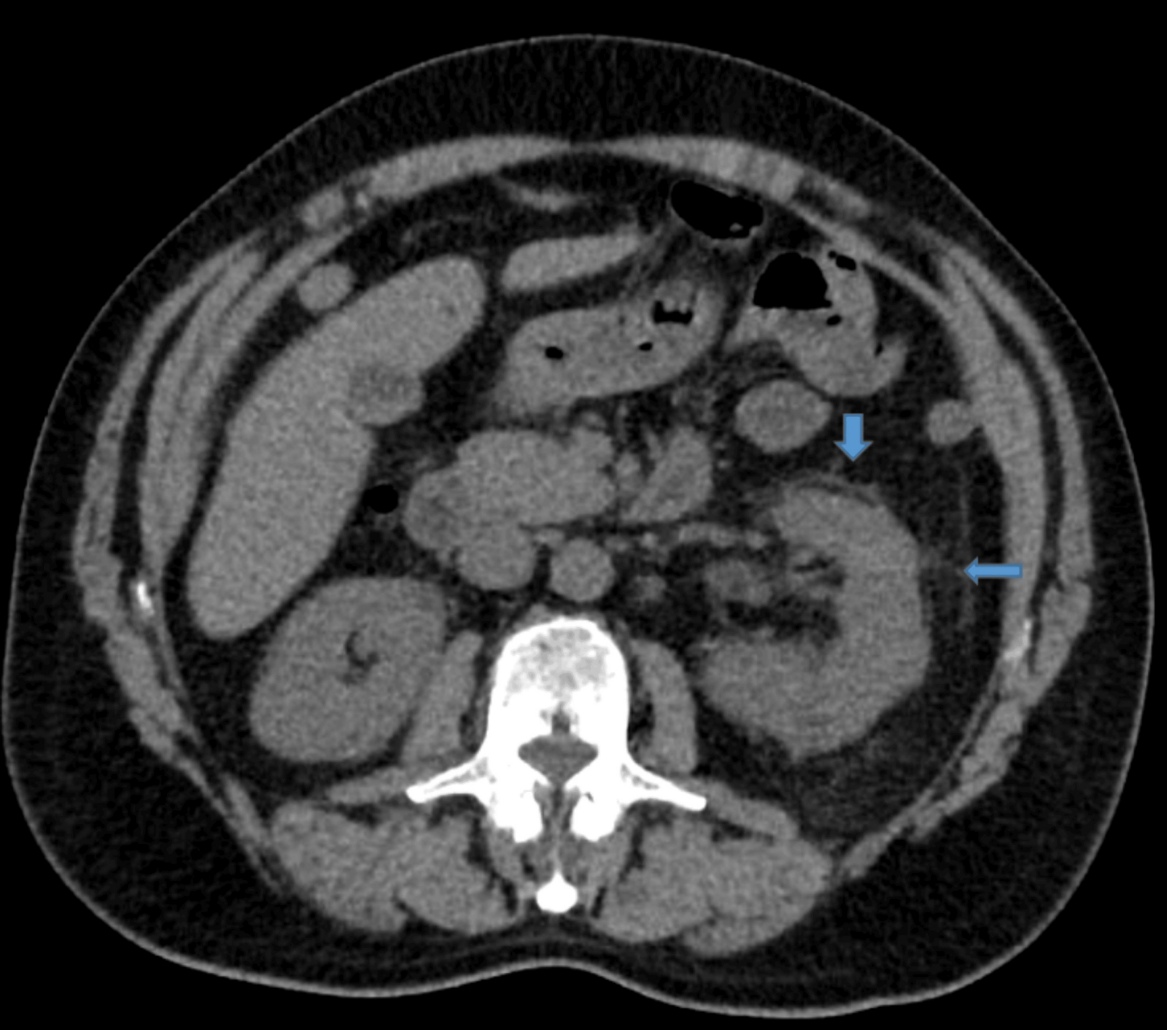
Computed tomography of the abdomen and pelvis without contrast showed left perinephric edema (blue arrow), consistent with left-sided pyelonephritis.
